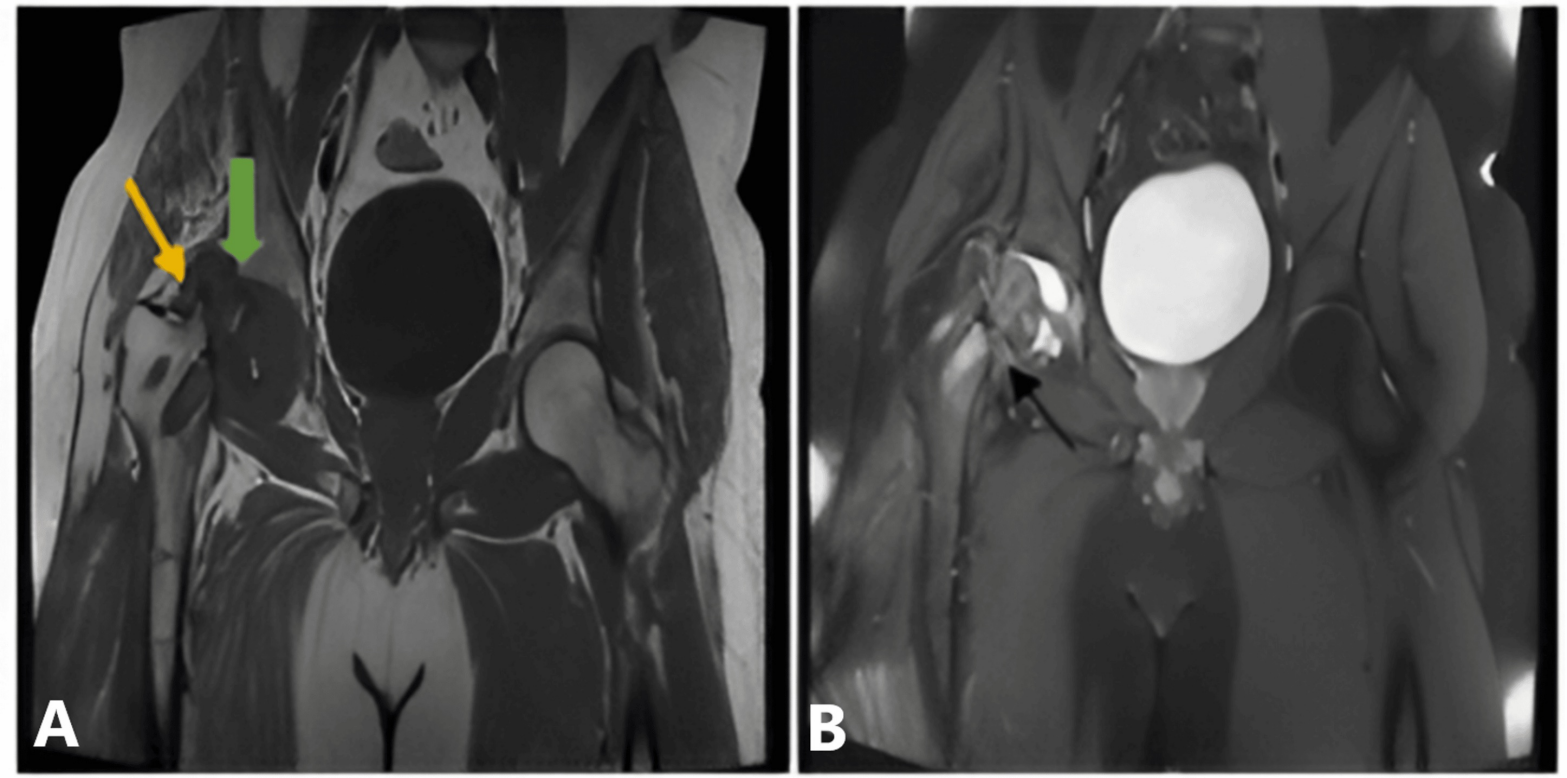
MRI of the pelvis and both hips. Findings:T1W axial and coronal images reveal a fracture of the neck of the right femur (yellow arrow), with signs of previous operative intervention; subluxation at the right hip joint; synovial effusion in the right hip joint; right femoral head AVN involving 70%-80% femoral head circumference (green arrow), with secondary degenerative changes; and Ficat and Arlet stage IV (green arrow) and ARCO stage IV (A). Coronal STIR images reveal significant hyperintense bone marrow edema in the right femoral head, neck, and proximal shaft (B; black arrow). T1W, T1-weighted; AVN, avascular necrosis; ARCO, Association Research Circulation Osseous; MRI, magnetic resonance imaging
